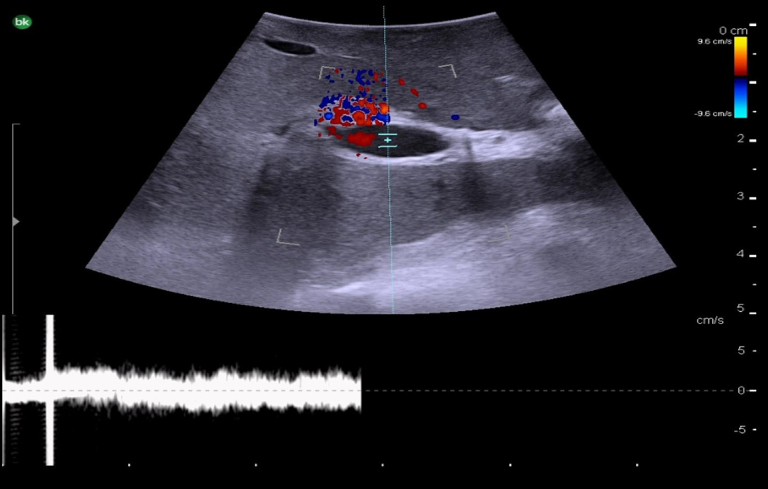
Intraoperative Doppler ultrasound of portal venous flow.
